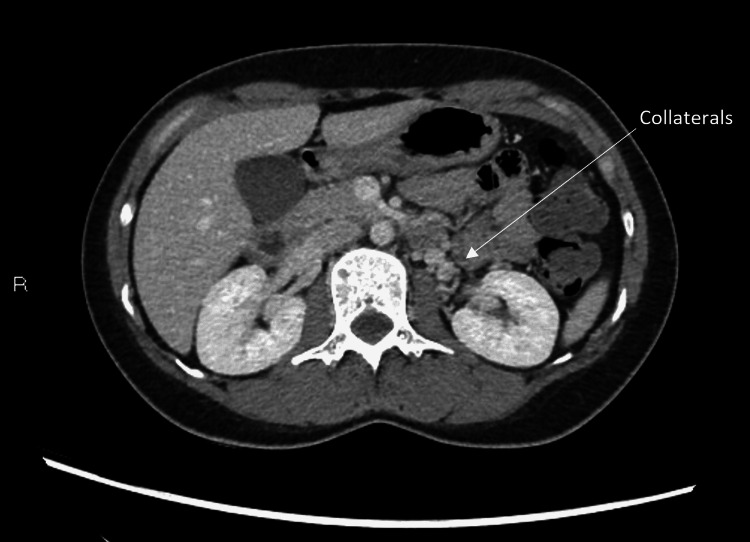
Axial CT images reveal multiple perinephric venous collaterals around the left kidney
